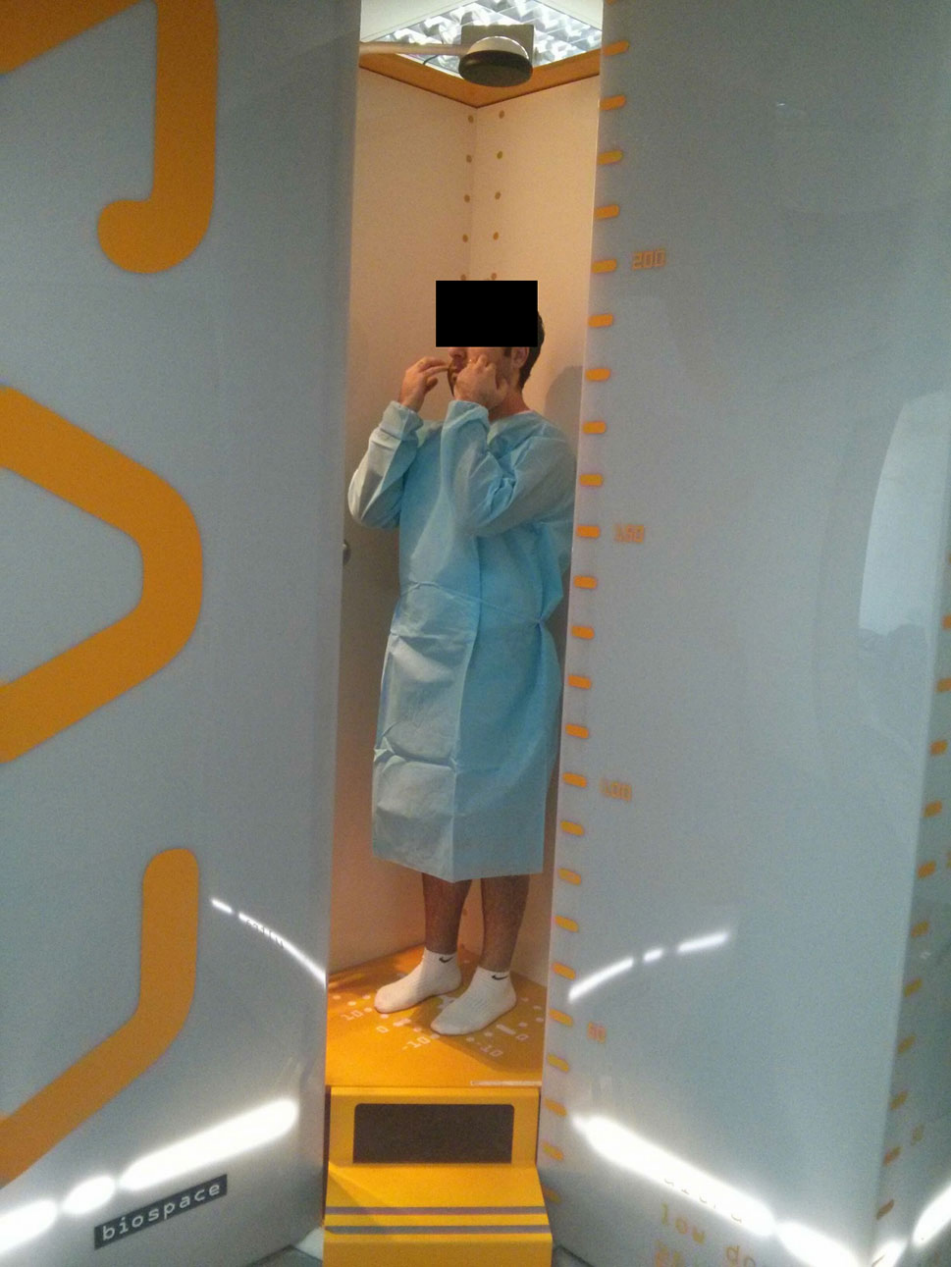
A patient standing in the EOS^®^ cabin in the required position for acquisition of orthogonal radiographs
